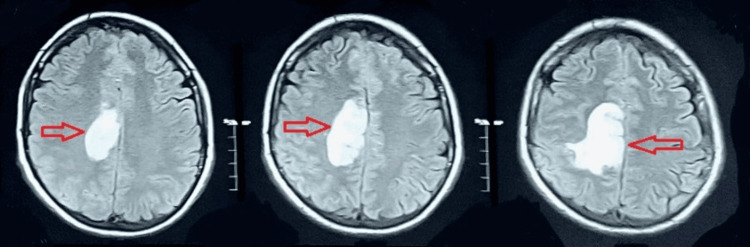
FLAIR MRI Bbrain A hyperintense lesion in the parietal region on FLAIR MRI. FLAIR: Fluid-attenuated inversion recovery
